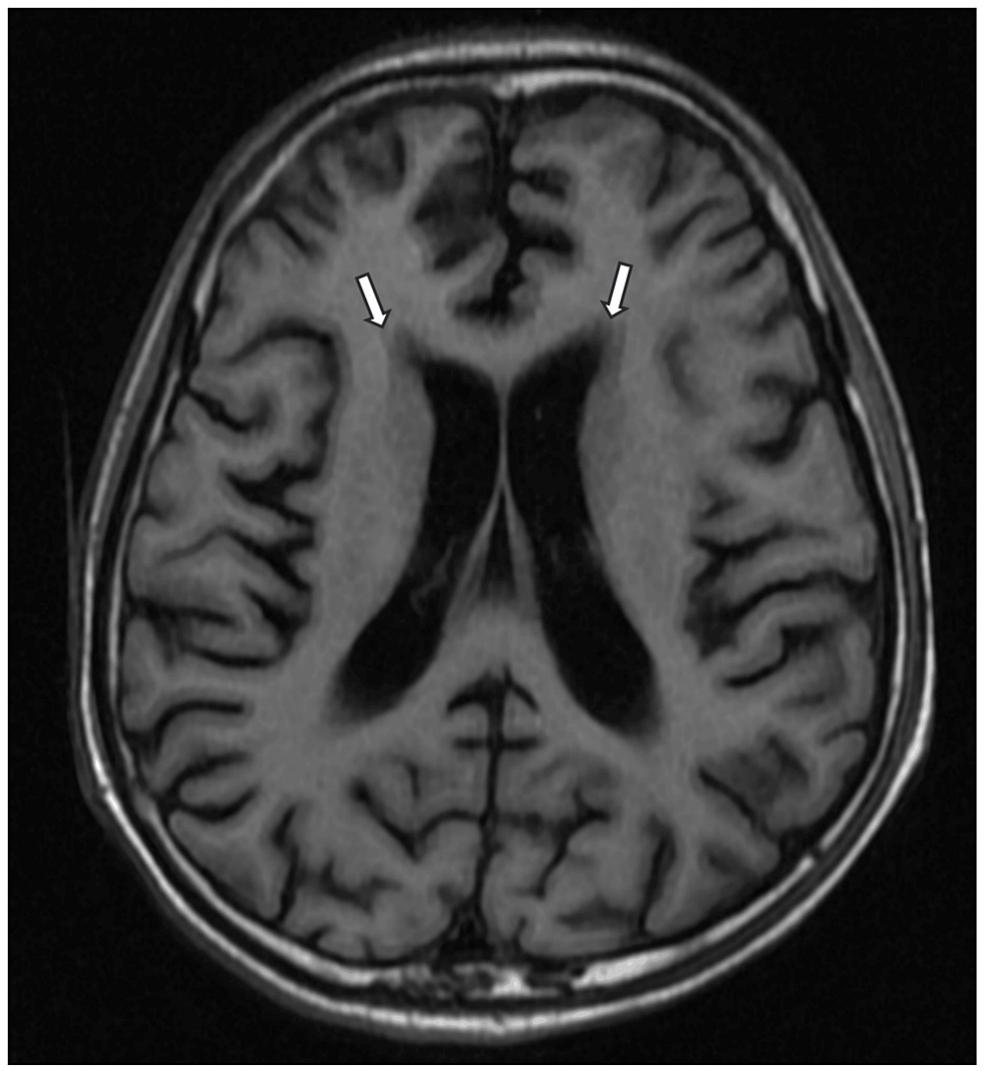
T1-weighted MRI brain (axial section) reveals diffuse periventricular white matter hypointensities (white arrow) MRI: magnetic resonance imaging
